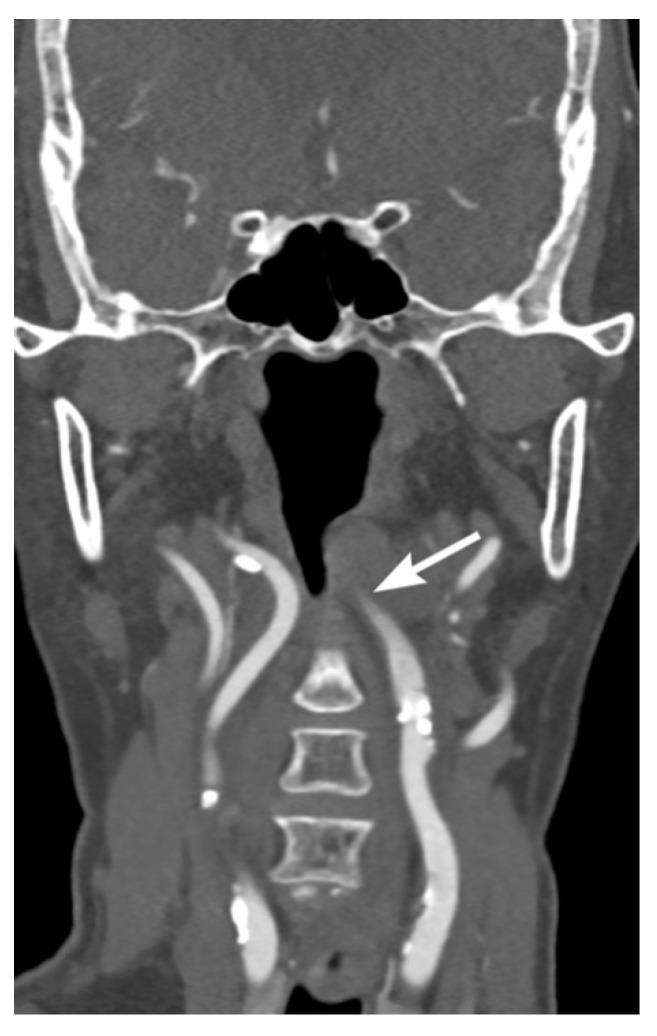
Long segment left internal artery occlusion in a 70-year-old male patient brought in by ambulance with multiple injuries following a motor vehicle accident. Coronal multidetector CT angiographic image shows tapering occlusion of the left internal carotid artery.
